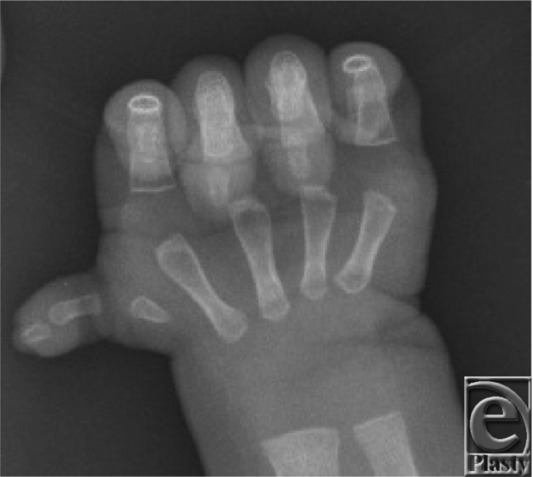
Radiograph of N.D. preoperatively, demonstrating thumb hypoplasia and an unstable carpometacarpal joint.
